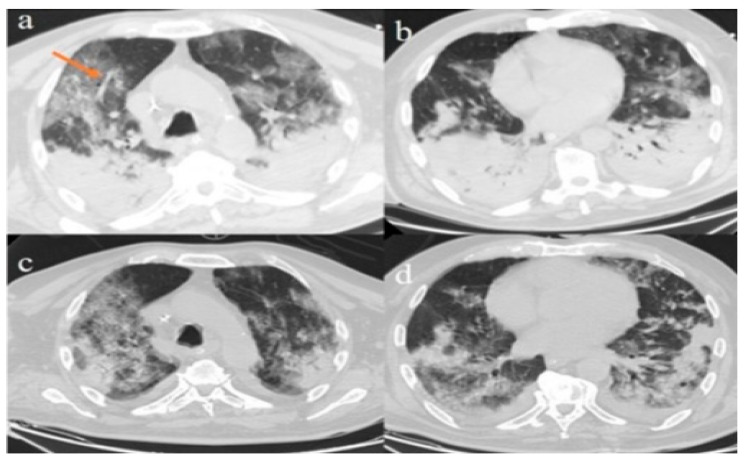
Chest CT of Case 2. Lung window (W: 1500 UH, L: −400 UH) of chest CT scan: ground-glass opacities in a crazy paving pattern with consolidations in the superior lobes with more posterior and peripheral distributions associated with severe consolidations in the inferior lobes (a,b) and vascular enlargement (orange arrow) (a); reduction of consolidations in the superior (c) and inferior lobes (d) after tocilizumab therapy.
